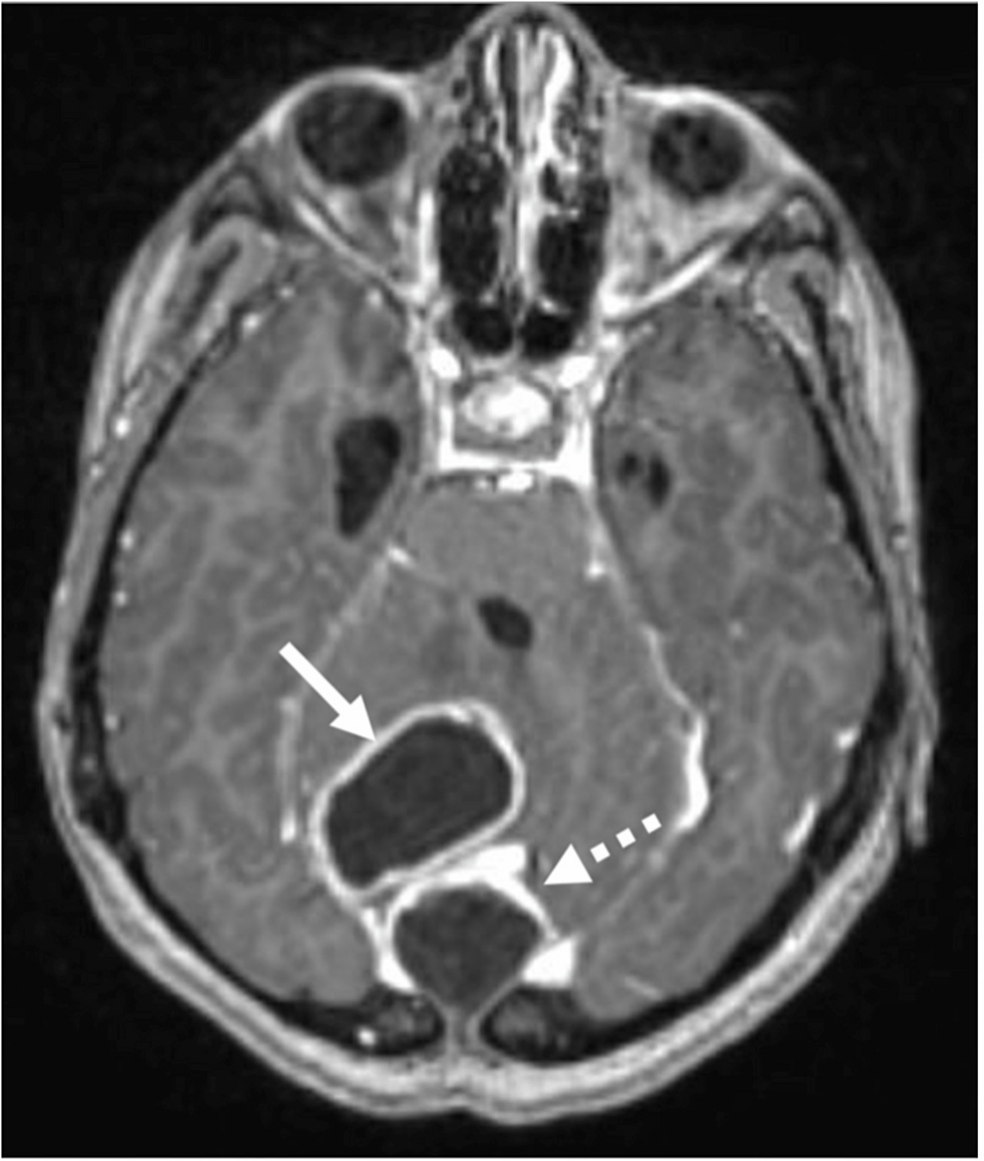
Postcontrast T1WI showing entire smooth peripheral enhancement of the main and daughter cysts (short and dashed arrows). T1WI, T1-weighted image
